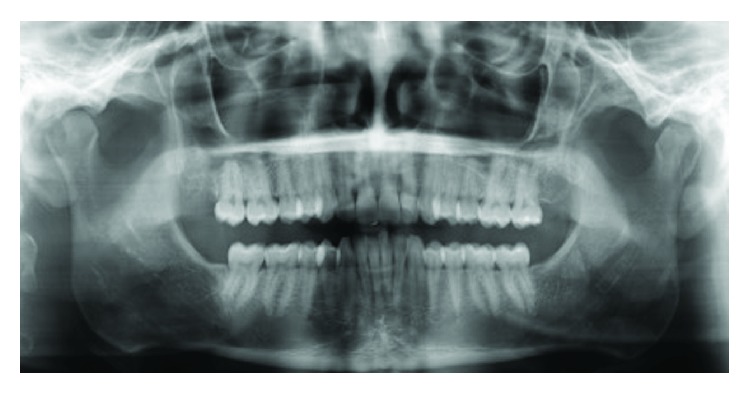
Panoramic radiography (one year postoperative).
